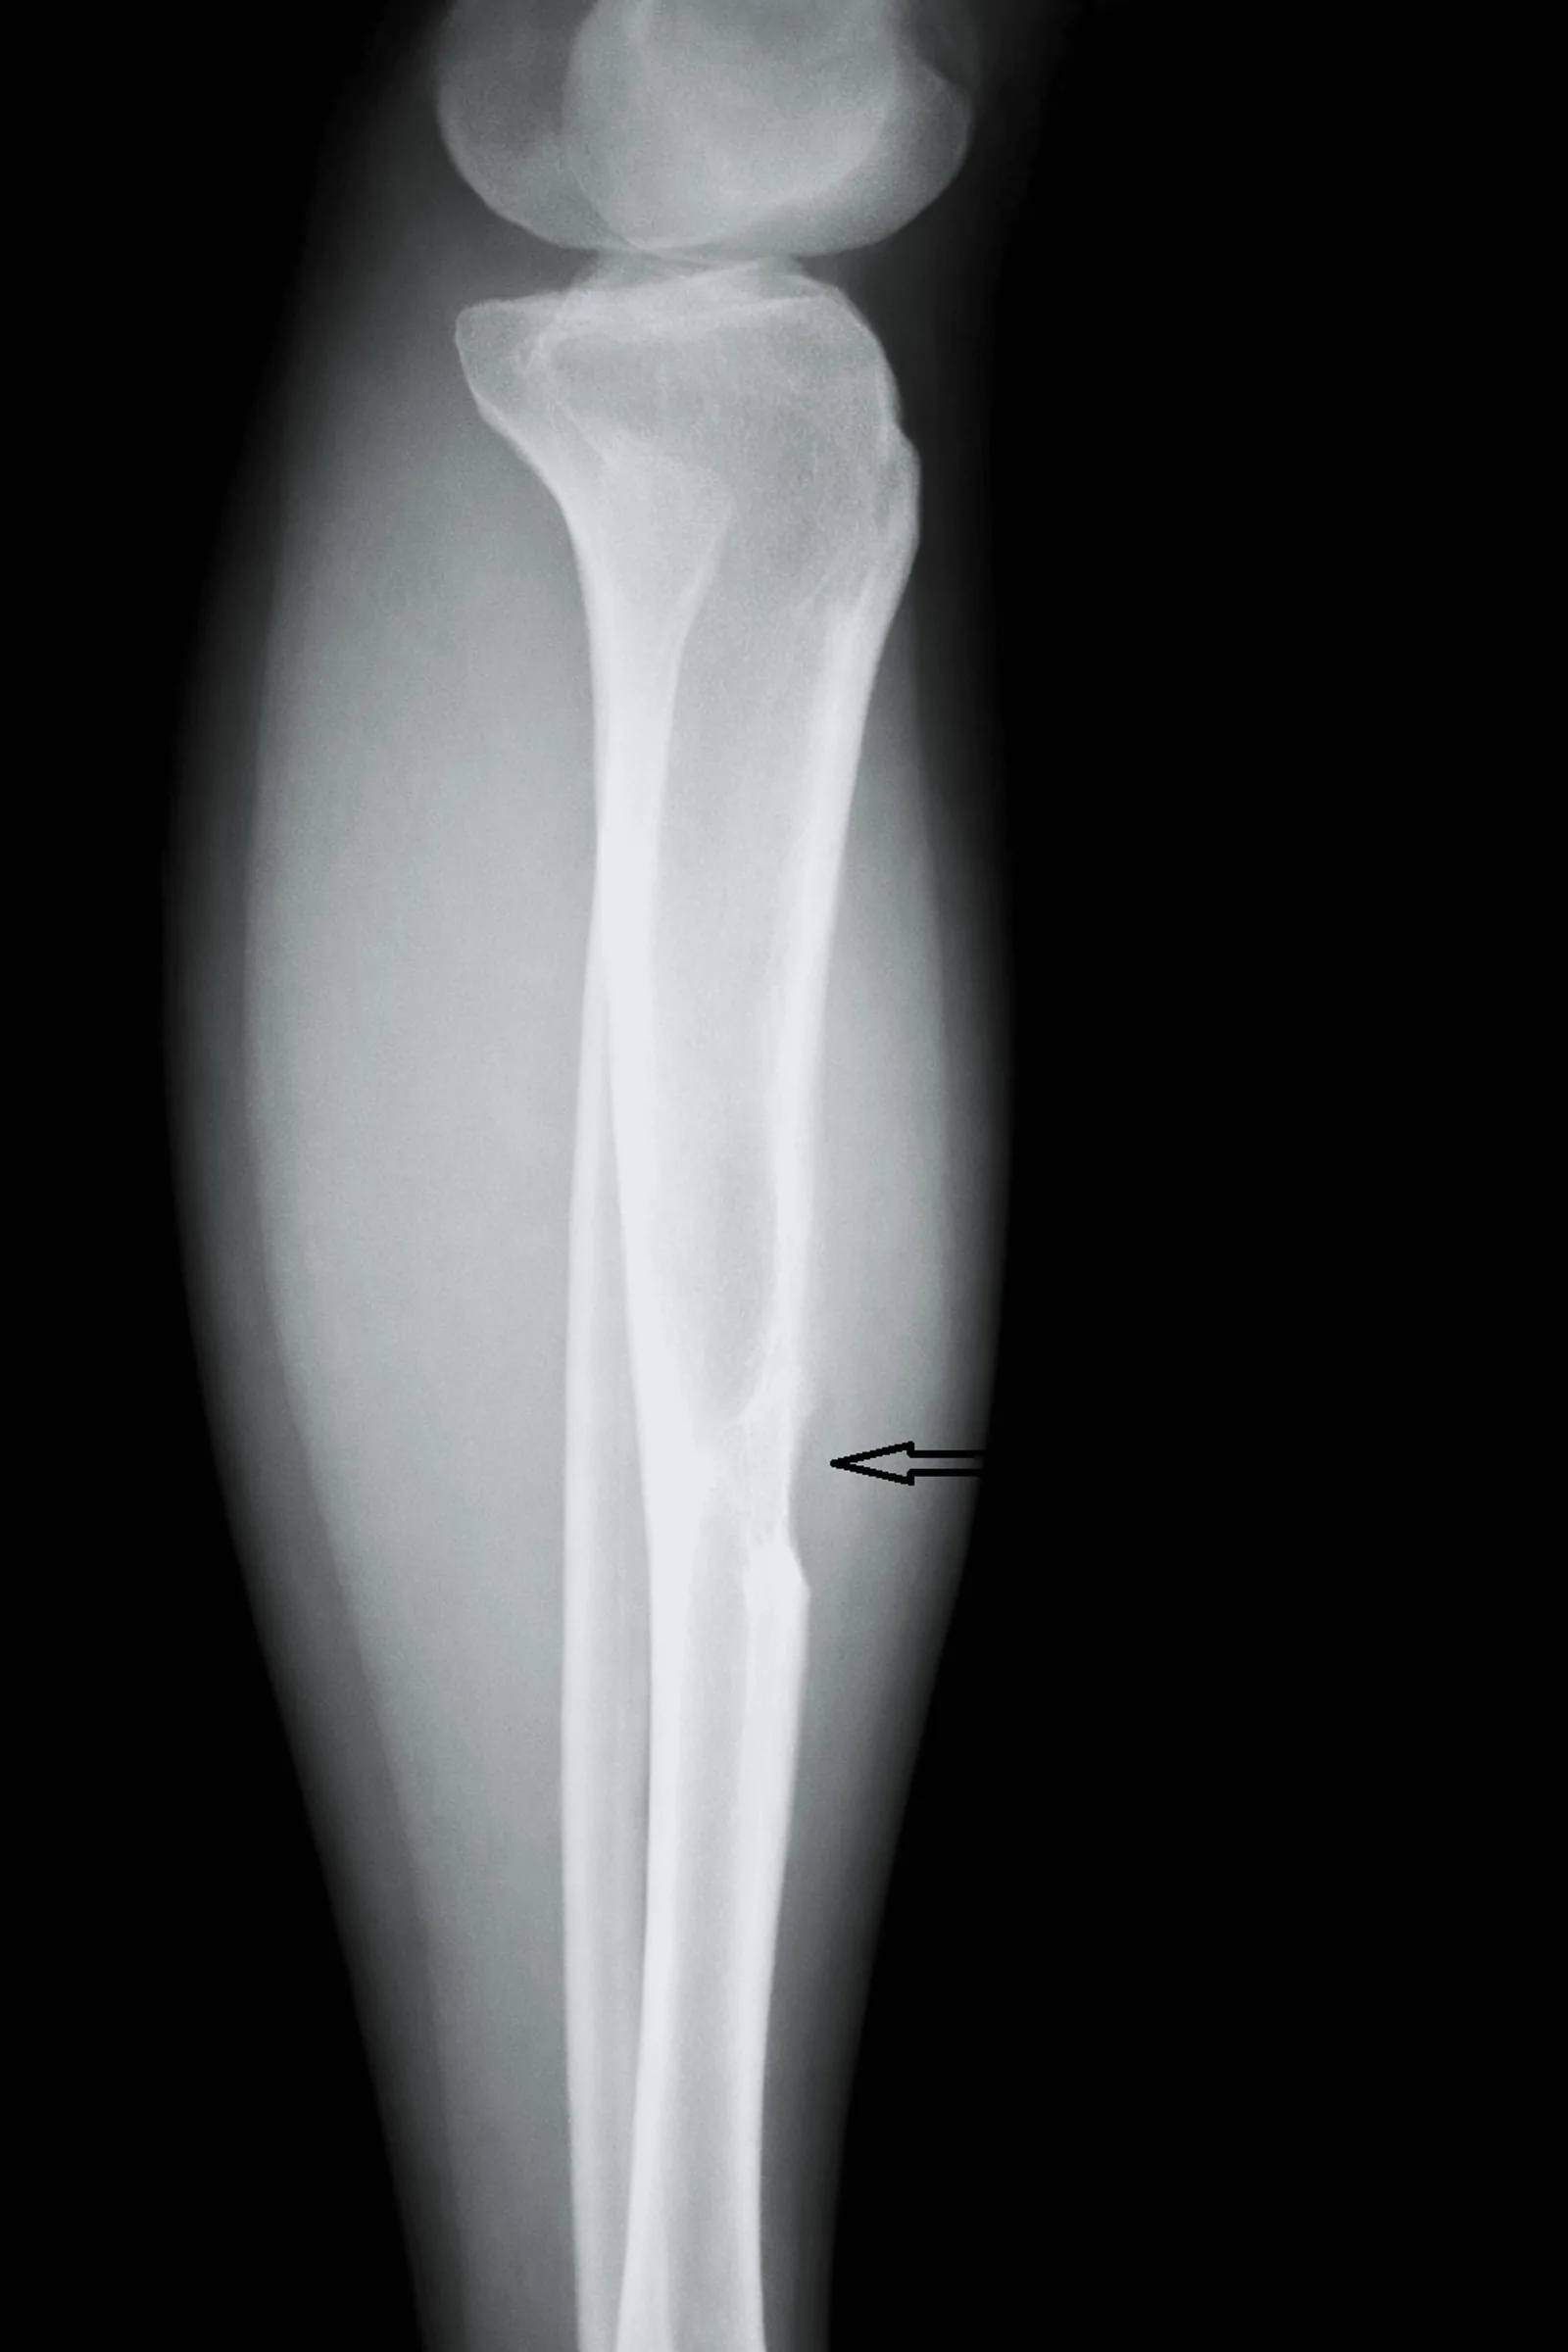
Lateral radiograph of the left tibia demonstrating adamantinoma. The radiograph displays the lateral aspect of the adult tibia and fibula. The black arrow points to an area of cortical irregularity and localized radiolucency on the anterior surface of the tibial shaft, consistent with adamantinoma. A soft tissue shadow of the leg is visible. Note the distinct appearance of the anterior cortical involvement and subtle bone changes within the diaphysis. Source: Original image owned by the authors.
